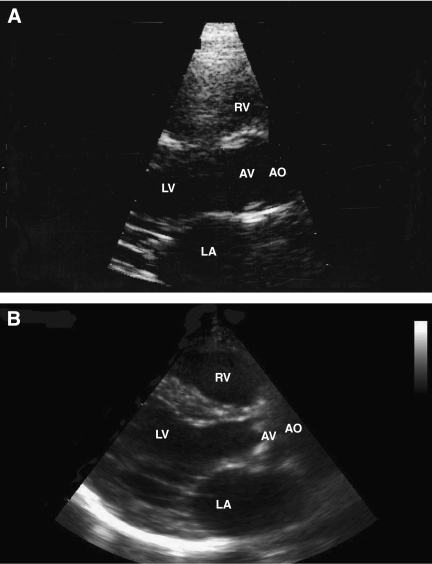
(A) In 1993 (7.5 years postradiotherapy), the aortic valve was without pathology and opened completely . (B) In 2007 (21.5 years postradiotherapy), echocardiography showed a thickened aortic valve, which hardly opened during ventricular systole in a patient with severe aortic stenosis. Abbreviations: AO, aorta; AV, aortic valve; LV, left ventricle; LA, left atrium; RV, right ventricle.
